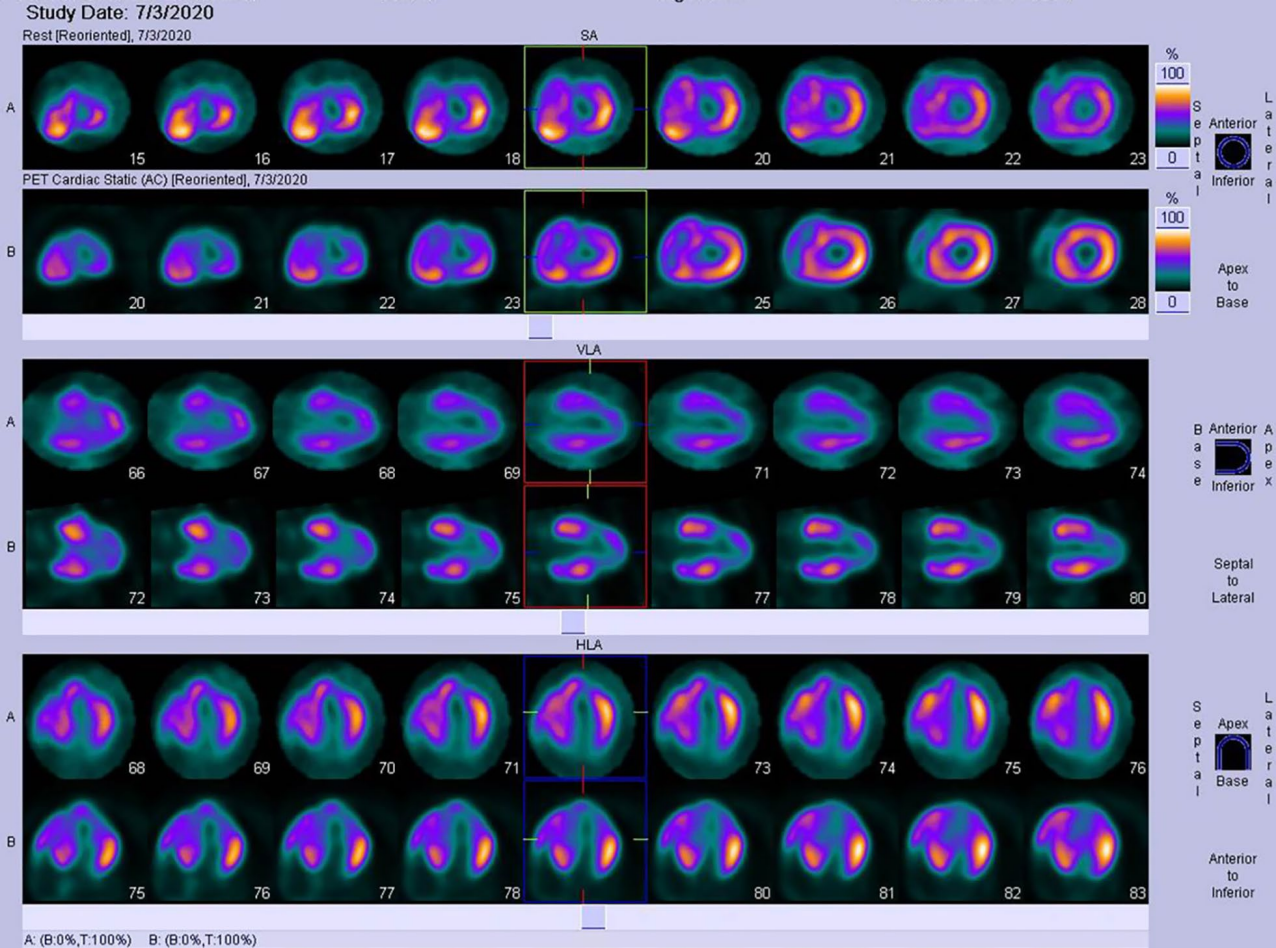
Positron emission computed tomography (PET-CT). Cardiac PET-CT showed that the myocardial infarction area was in the apex and left ventricular anterior wall
